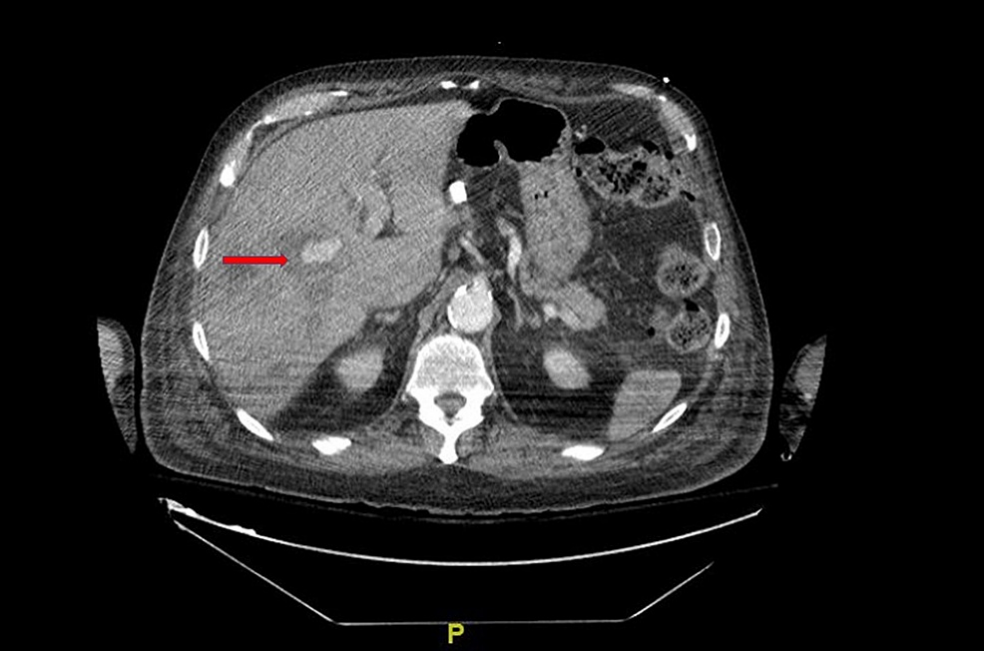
Axial view hepatic artery pseudoaneurysm seen on CTA abdomen Red arrow pointing to the hepatic artery pseudoaneurysm as seen on an axial view on CTA abdomen. computed tomography angiography (CTA)
